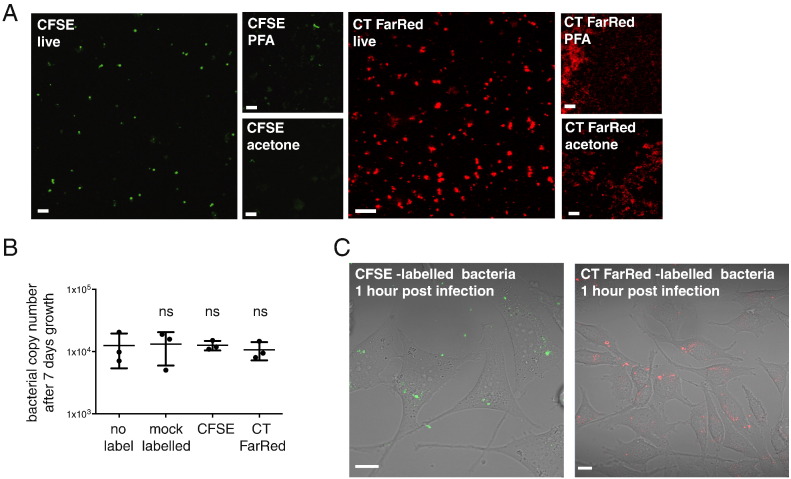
(A) Purified bacteria labelled with CFSE (left panels, green) or CellTrace FarRed (right, red) and imaged live or fixed with paraformaldehyde (PFA) or acetone prior to imaging. (B) Graph showing the bacterial copy number in one well of a 24-well culture plate after 7 days growth in the presence or absence of CFSE/CT FarRed. Inoculum used was 1.36 × 10^4^ ± 1.9 × 10^3^ bacteria per well (mean ± SD). Individual replicate values are plotted and the mean ± SD is shown. The results of the one-way ANOVA statistical test using the no label condition as a comparison are shown. (C) Images of live L929 cells infected with CFSE or CT FarRed-labelled bacteria. Scale bar = 10 μm. (For interpretation of the references to colour in this figure legend, the reader is referred to the web version of this article.)
